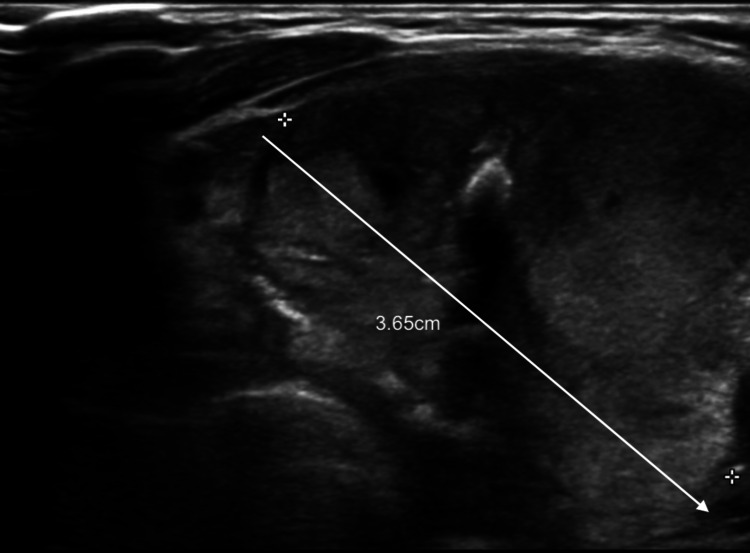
USS showing a 3.65cm U4 left thyroid nodule lesion (i.e., a suspicious thyroid nodule). USS - ultrasound
